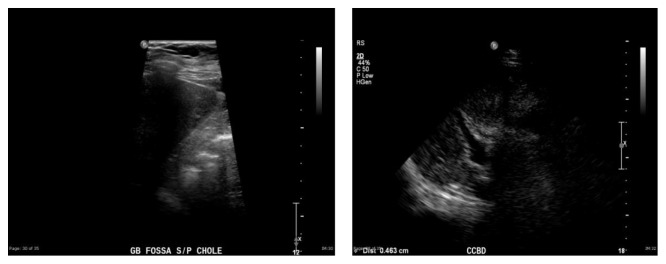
Postoperative abdominal ultrasound showing no evidence of collection and distal common bile duct diameter of 4.6 mm.
